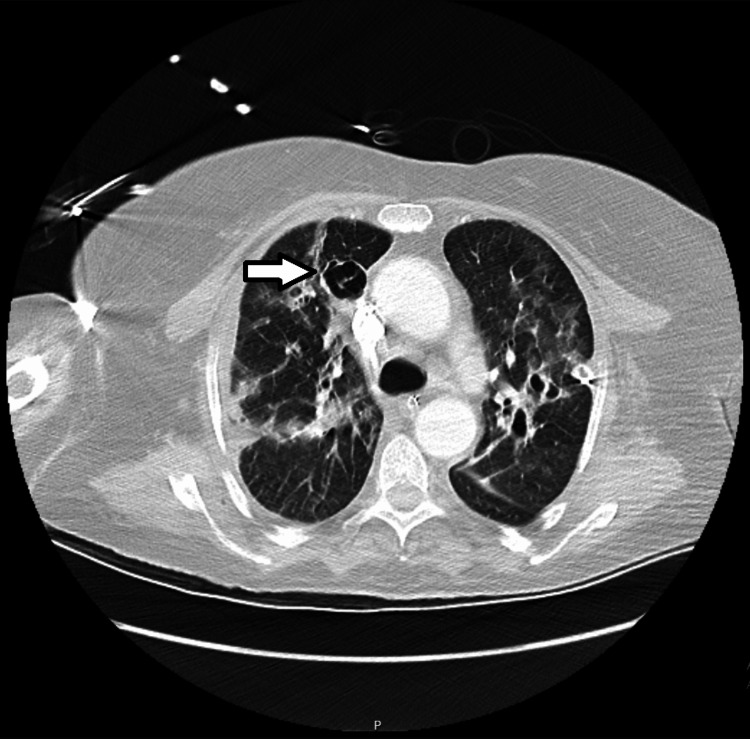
Computed tomographic scan of the thorax on the 20th day in the intensive care unit Diffuse bilateral subpleural ground-glass opacities with fibrotic components and necrotizing bilateral pneumonia (arrow) are evident.
